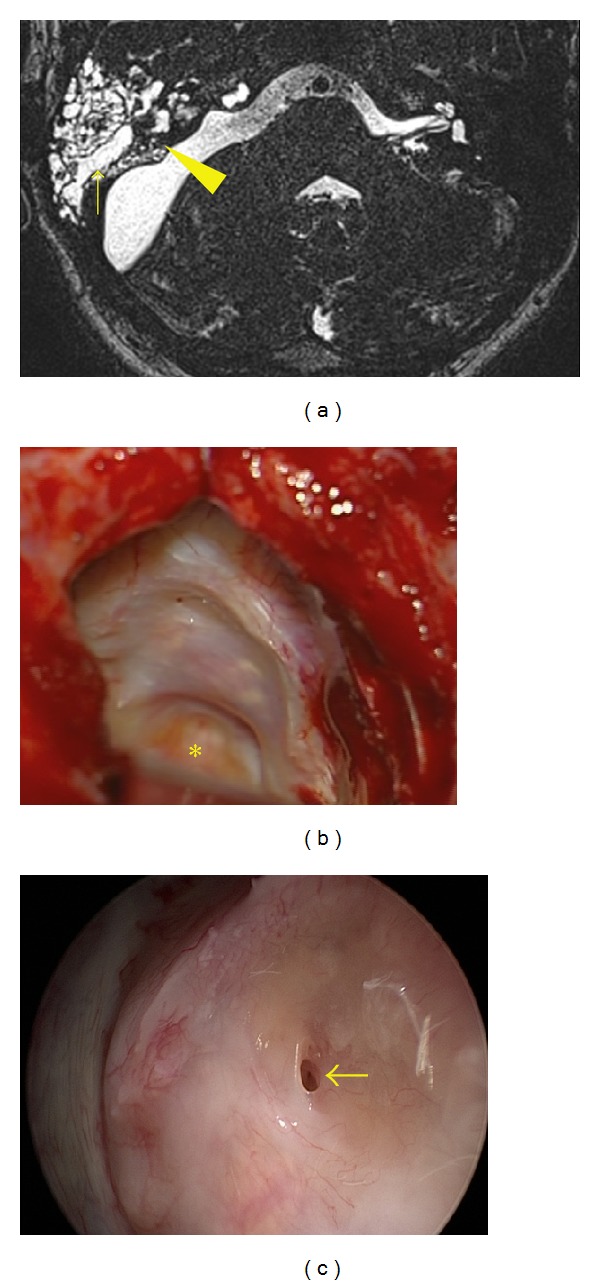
Medial variant of CSF leak. (a) T2W MRI, arrowhead pointing to the fistula; arrow demonstrates CSF filled pneumatic system of temporal bone. (b) Wound revision with identification of fistula in the posterior rim of meatotomy; asterisk shows the closed IAC. (c) Endoscopic view of the fistula.
